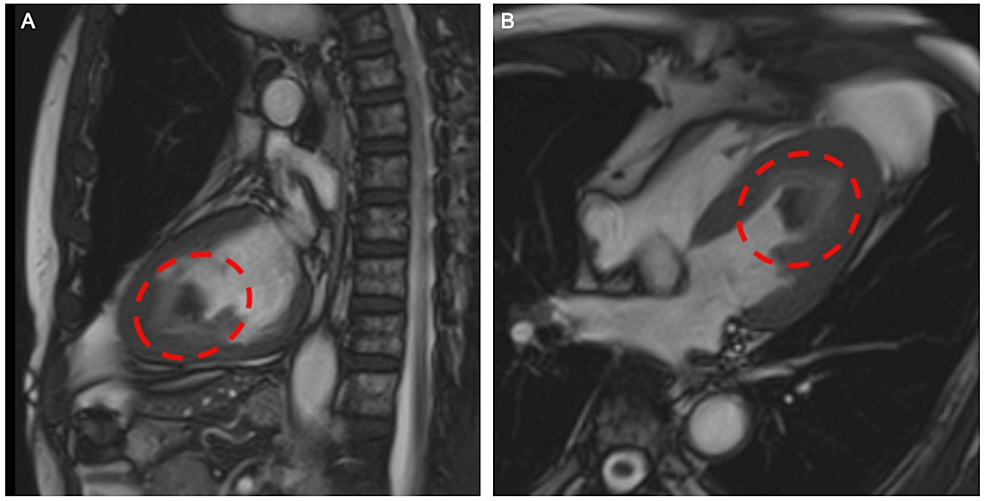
Cardiac MR image The red circle indicates the thrombosis in the sagittal (A) and horizontal (B) sections. A mobile mass with a low signal density on T1WI and T2WI was observed in the apex of the left ventricle; dynamic contrast showed no contrast effect.
